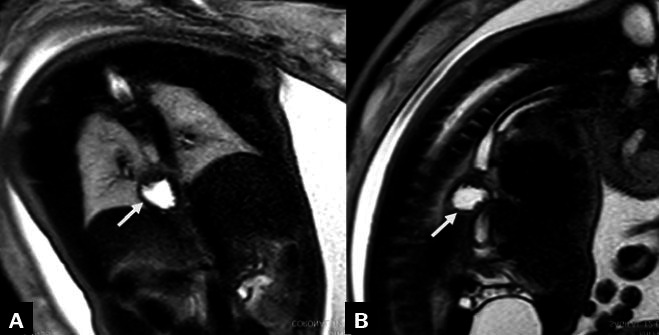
Two-dimensional ultrasound images, acquired with a convex 3.5 MHz
